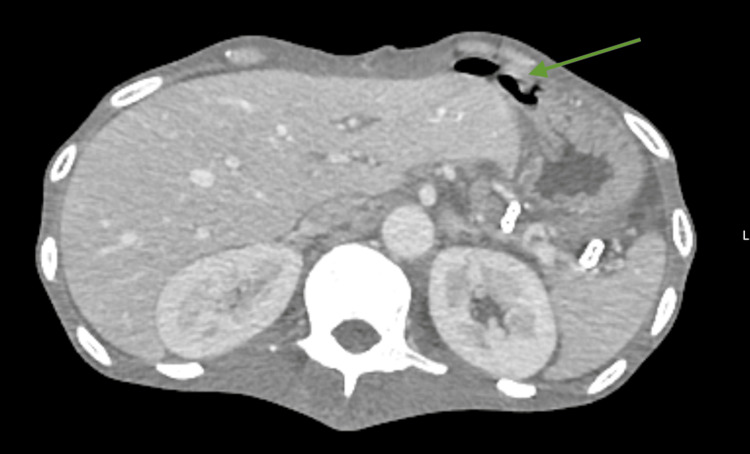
Transverse plane of the abdomen on a the contrast enhanced CT showing the pneumoperitoneum in the upper quadrants Green arrow: pneumoperitoneum in the upper quadrants of the abdomen.
